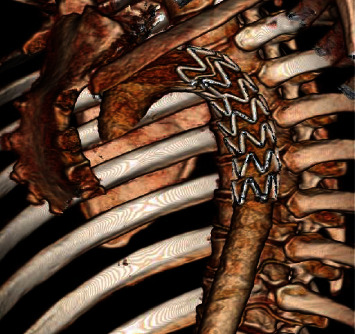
Postoperative CT image reconstruction demonstrating graft location and the successful occlusion of the pseudoaneurysm.
